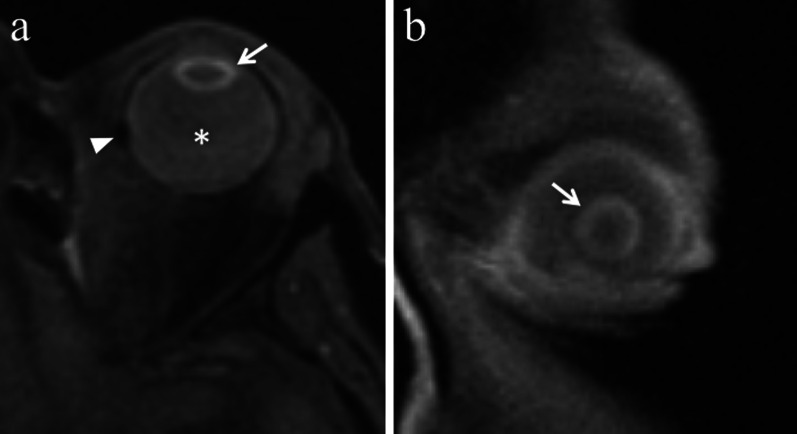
A 65-year-old man with radiation-induced cataract after proton-beam radiotherapy for choroidal melanoma of the left eye. a Axial and b coronal fat-supressed T1-weighted images show a subcapsular peripheral hyperintense rim of the left lens (white arrows), consistent with radiation-induced cataract. Note the slight hyperintensity of the vitreous body (white asterisk in a) due to intraocular inflammation. A small metal artefact (white arrowhead in a), due tantalum clip, is detectable along the medial aspect of the sclera
